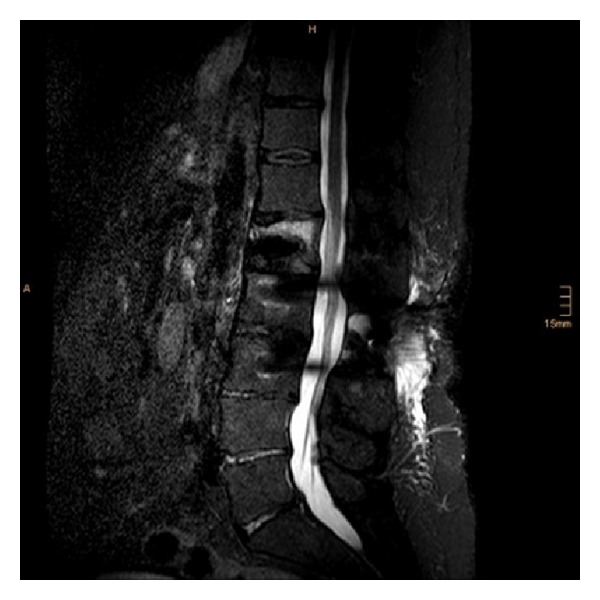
Postoperative sagittal MRI (STIR).
